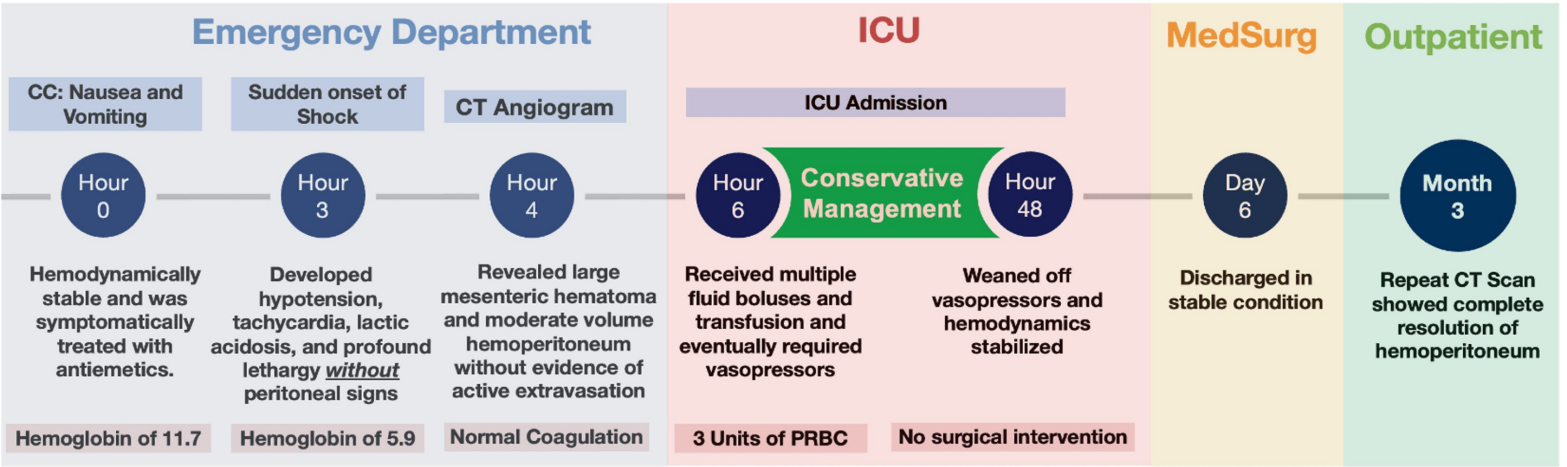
Timeline of non-operative management in idiopathic spontaneous intraperitoneal hemorrhage. The patient presented with nausea and vomiting and later developed hemorrhagic shock. CT imaging revealed a large mesenteric hematoma with moderate hemoperitoneum but no active bleeding. Managed conservatively in the ICU with transfusions and vasopressors, the patient stabilized without surgical intervention and was discharged on day 6. Follow-up imaging at three months confirmed complete resolution CT: computed tomography; ICU: intensive care unit; PRBC: packed red blood cells
